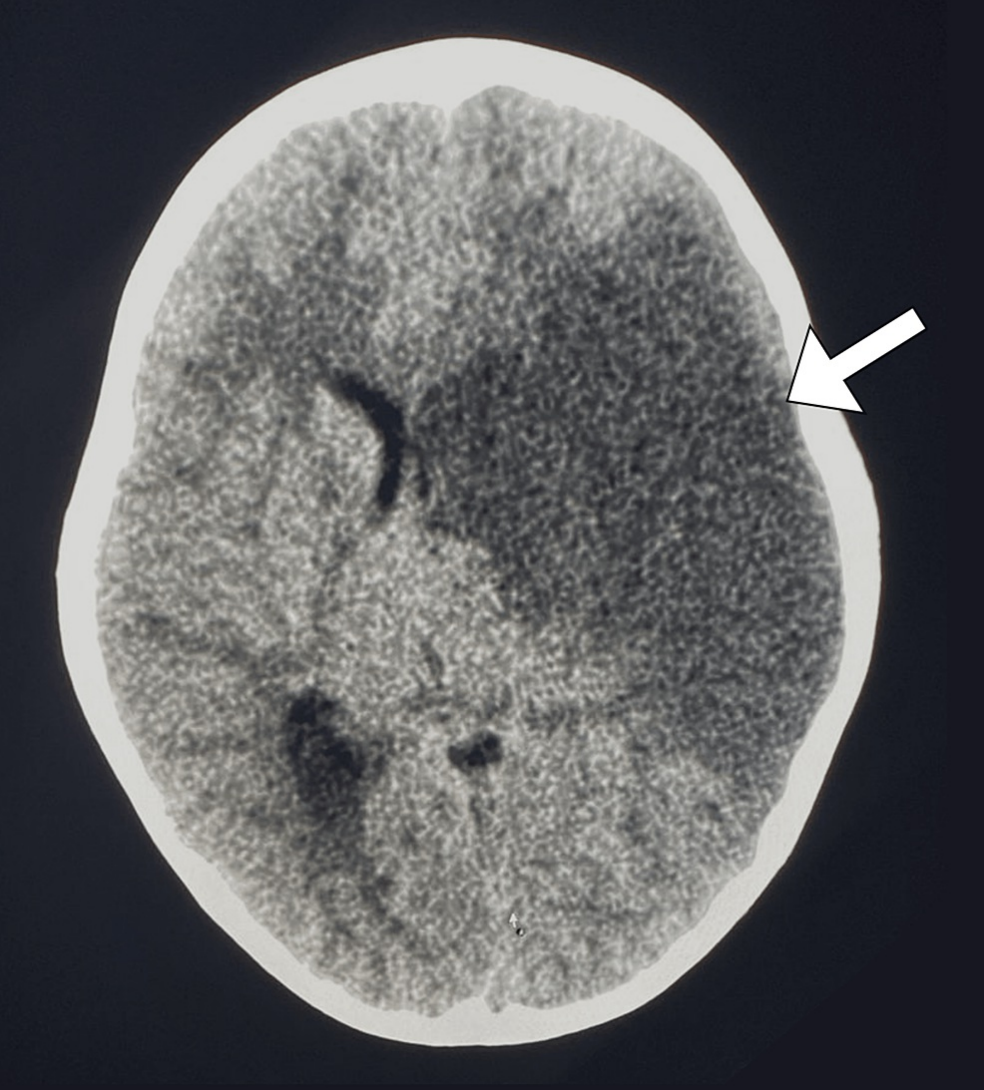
Axial CT image showing a large wedge-shaped hypodense area (arrow) in the left parietal lobe with associated loss of grey-white matter differentiation consistent with ischemic stroke CT: computed tomography
